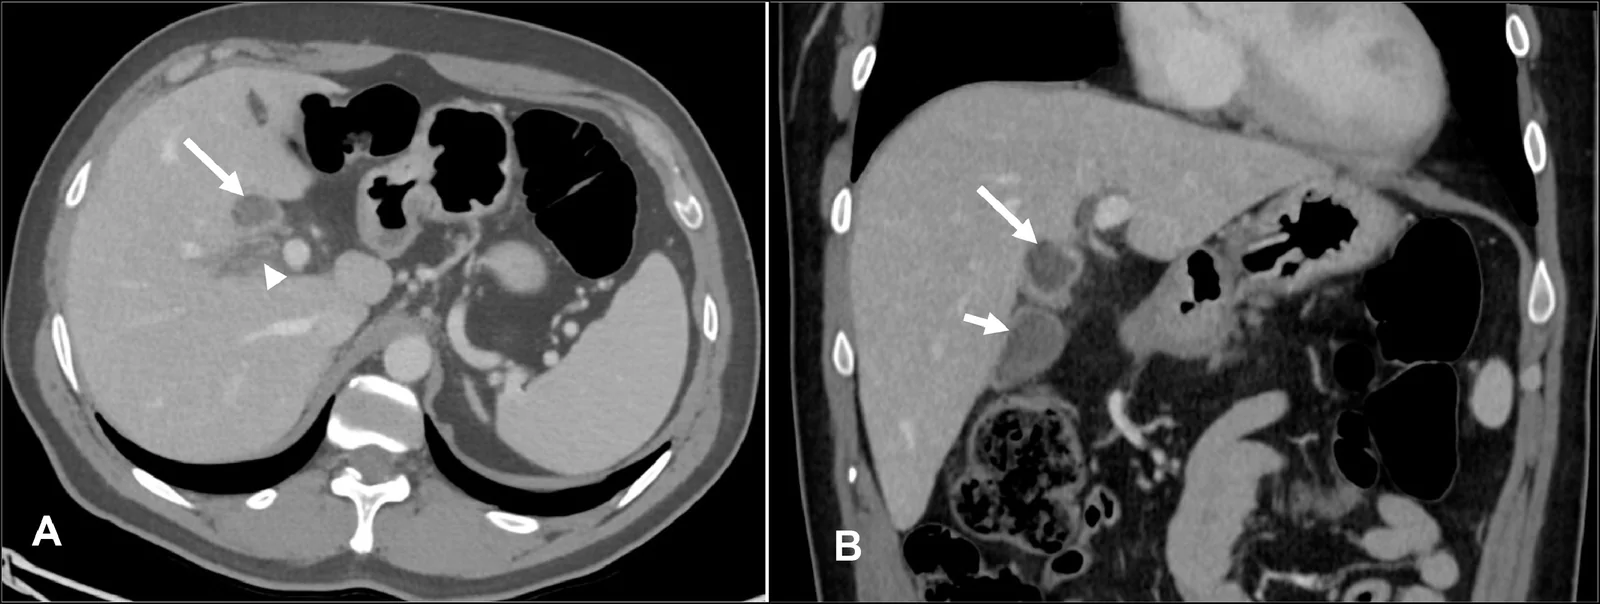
(A and B) Axial and coronal contrast-enhanced CT images demonstrate an ovoid structure, the accessory gallbladder (long white arrow) located superior to the orthotopic gallbladder (short arrow). The common bile duct is dilated, with wall thickening and enhancement, consistent with acute cholangitis (arrowhead).
